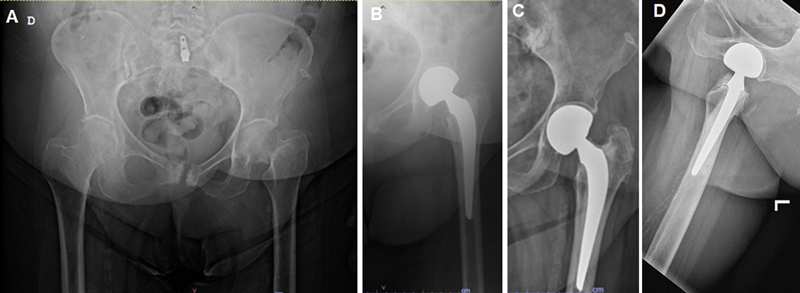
(A) Plain pelvic radiographs of an 82-year-old female showing left displaced femoral neck fracture. (B) Immediate postoperative left hip plain X-ray showing cemented bipolar hemiarthroplasty. (C, D) 16-month postoperative plain left hip radiographs showing acetabular erosion. The patient complained of groin pain with movement and was managed conservatively.
